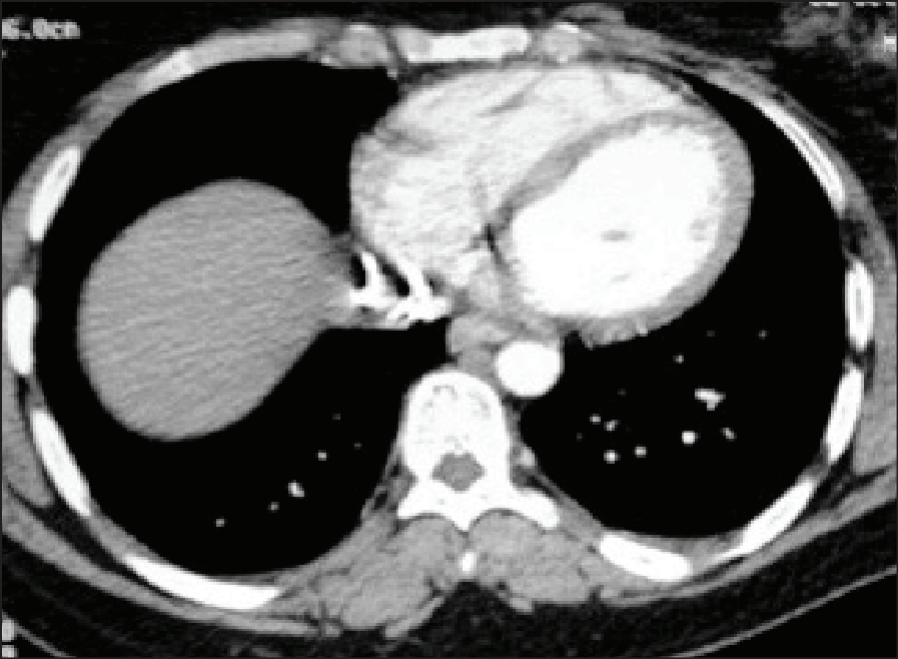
CT showing stent location in vena cava.
